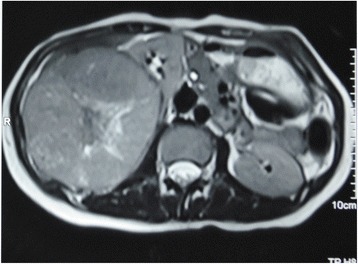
MR image showing mass in the right kidney
